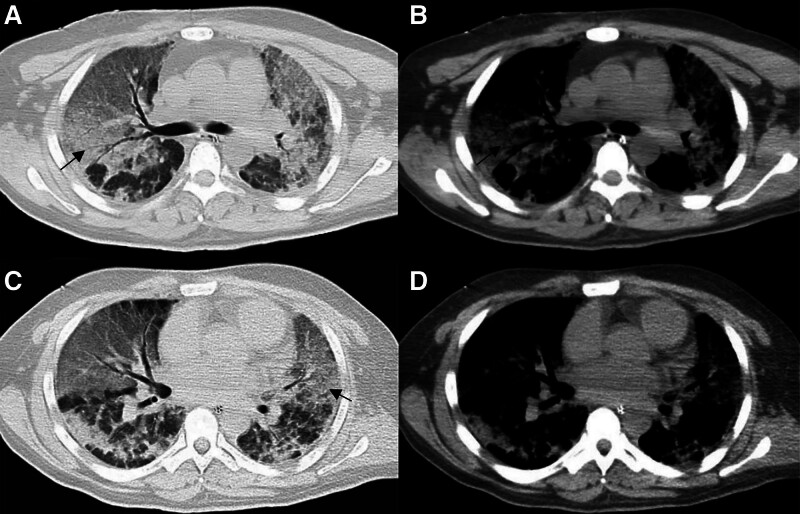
Computed tomography of the chest. (A and C) Chest computed tomography scans obtained with lung window settings. (B and D) Mediastinal window images of the chest computed tomography scan from the patient. The black arrows demonstrate extensive interstitial changes in both lungs.
